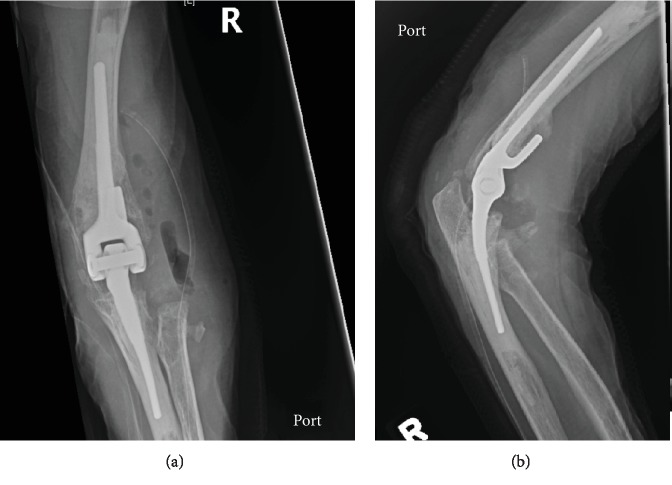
(a) AP and (b) lateral radiographs of R elbow in recovery bay following excision of HO.
